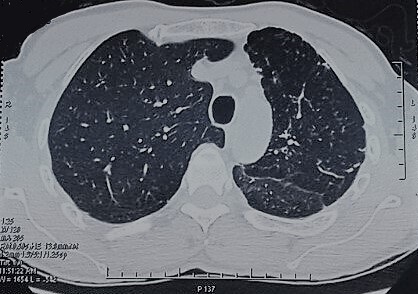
Chest CT parenchymal section: hernia of the right lung, reduction of the left lung volume with air trapping of the left upper lobe without intra-parenchymal or bronchial abnormality
